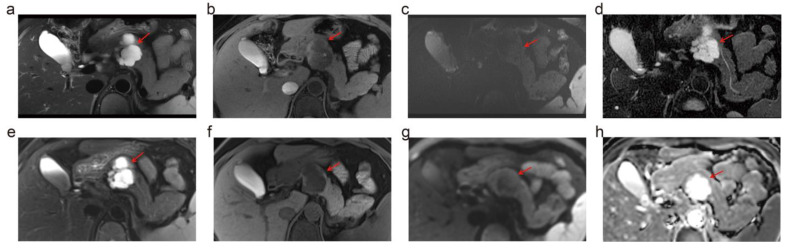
The images from 5.0 T and 3.0 T MRI of a 44-year-old female. This patient was suspected of serous cystadenoma, which was stable at imaging follow-up. 5.0 T MRI demonstrates a sharper contour of the lesion and more delicate details, including the thin septa within the lesion, the small central scar, and the relationship with the non-dilated main pancreatic duct, compared to the 3.0 T MRI. (a–d) show the T2-weighted imaging, T1-weighted imaging, DWI, and ADC map of 5.0 T MRI. (e–h) display the T2-weighted imaging, T1-weighted imaging, DWI, and ADC map of 3.0 T MRI. The red arrows refer to the lesion.
